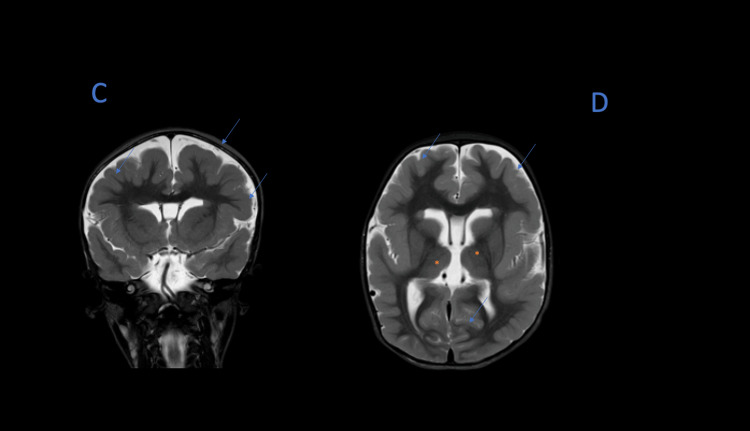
(C) MRI, T2 sequence, coronal view: Blue arrows show the polymicrogyria. (D) MRI, T1 sequence, sagital view: Blue arrows show the polymicrogyria and the orange asterix show the subependymal gray matter heterotopia MRI: magnetic resonance imaging
